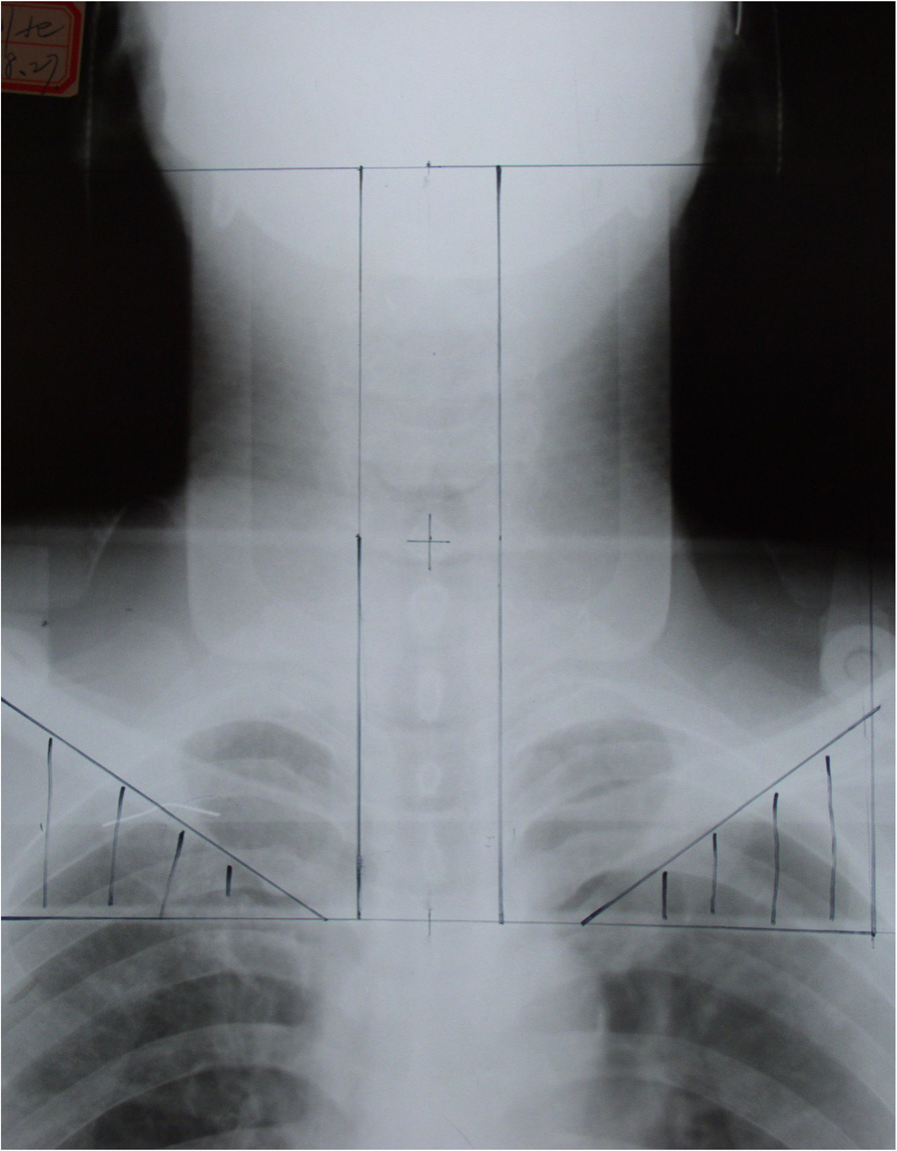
X-ray showing the delineation of the whole neck tangential field in the facial and cervical regions after 19 fractions of radiotherapy (38 Gy); the transferred submandibular gland is shielded.
